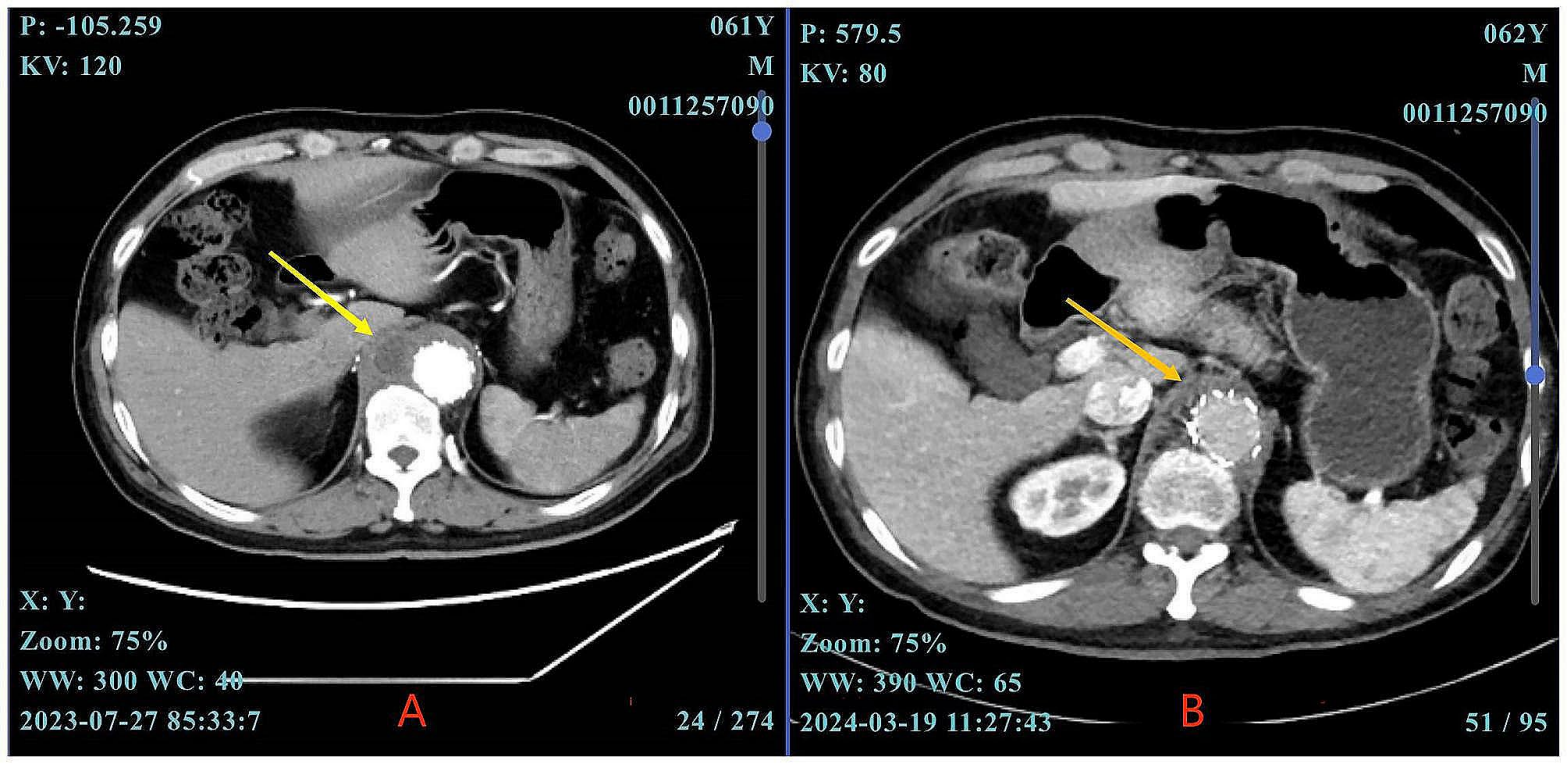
The abdominal CTA of case 2. (A) an annular low-density focus on the right side of the thoraco-abdominal aortic stent (yellow arrows). (B) a noticeable reduction in the size of the necrotic focus (orange arrows)
